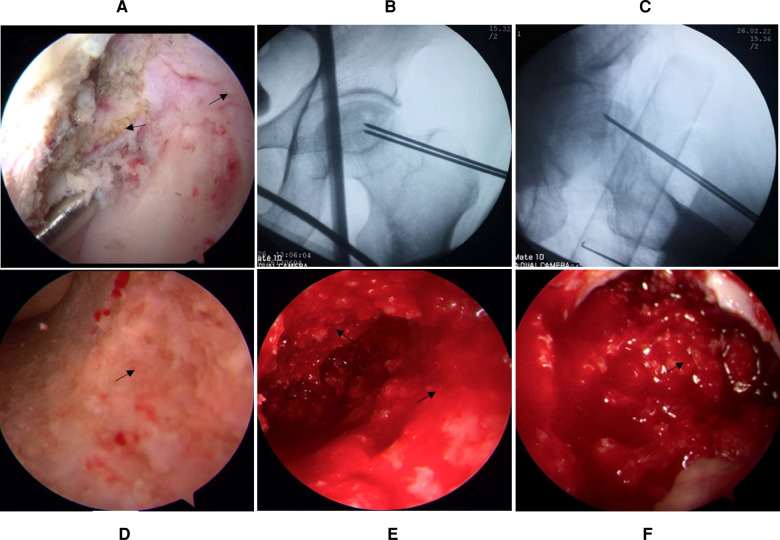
The capsule is cut longitudinally (the black arrow), and the necrotic site is located using a Kirschner pin under arthroscopic (A) and radiographic (B,C) visualization. (D) Appearance of the necrotic site before debridement. (E) Appearance of the necrotic site after debridement. (F) Arthroscopic appearance after bone particle grafting.
